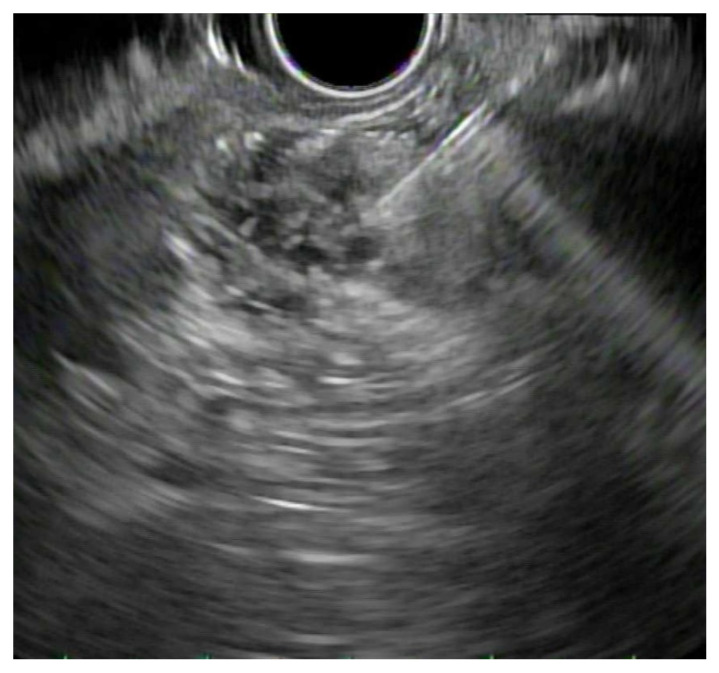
EUS Celiac Plexus Neurolysis (CPN): Endosonographic image of a celiac plexus neurolysis.
